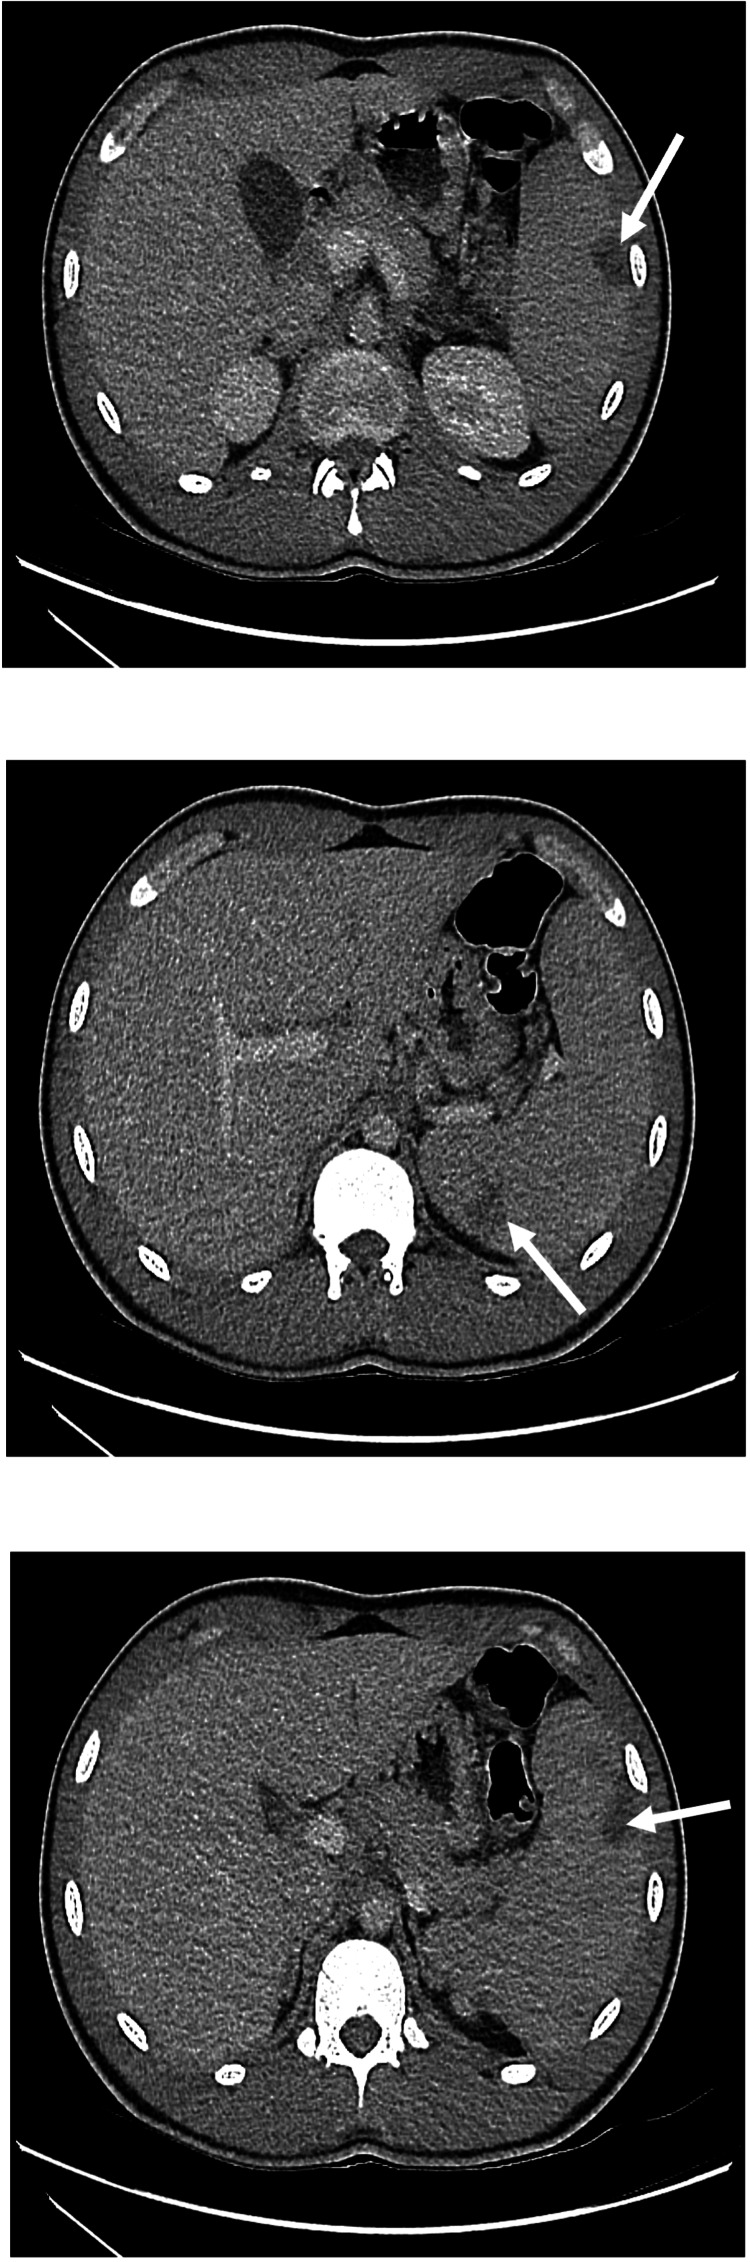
Cross-sectional imaging (axial view of computed tomography of the abdomen with intravenous contrast) demonstrating splenomegaly with hypoattenuated lesions indicative of splenic infarcts in the patient described (white arrows)
